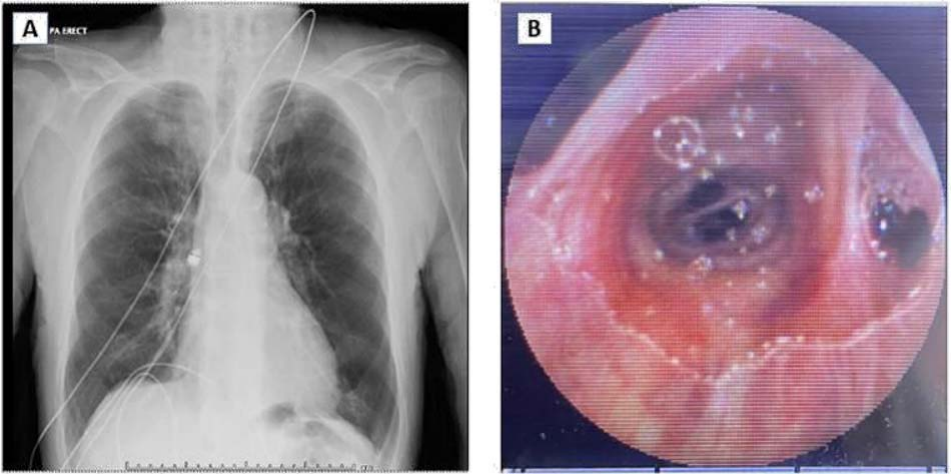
(A) Chest X-ray showing capsule in the right main bronchus. (B) Capsule lodged in bronchus intermedius showing endobronchial anatomy.
